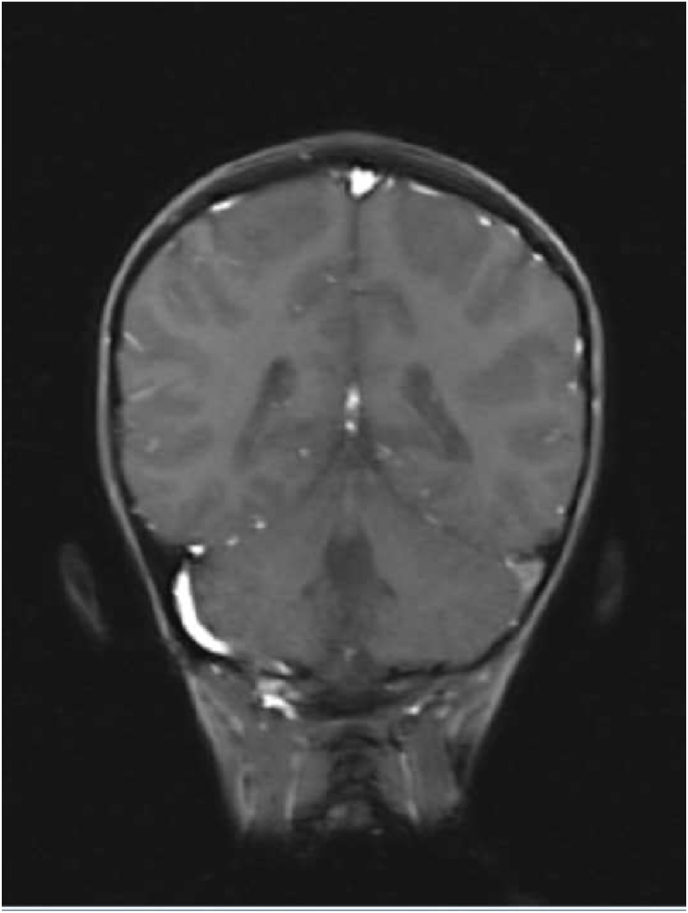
Coronal T1-weighted post-contrast images confirm filing defects within left transverse sinus and internal jugular vein.
